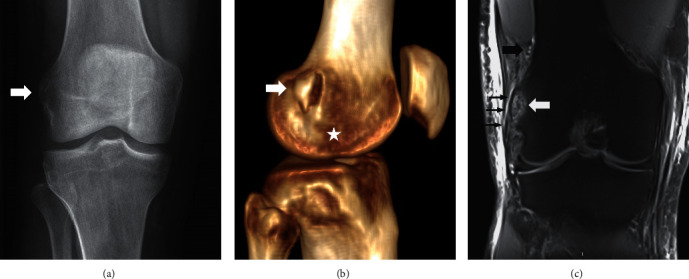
Patient case 2. (a) AP right knee X-ray at day 1 showing the avulsion fracture of the femoral insertion of the LCL (white arrow). (b) Lateral view of a 3D reconstruction of the right knee CT at day 1 showing the avulsed bony fragment of the femoral insertion of the LCL (white arrow). The white star shows insertion site of the popliteal tendon. (c) Proton density coronal MRI obtained at day 3 showing an intact LCL (small black arrows) attached to the avulsed bony fragment of the femoral insertion (large white arrow). Note the superior lateral genicular vessels (large black arrow) above the bony fragment.
